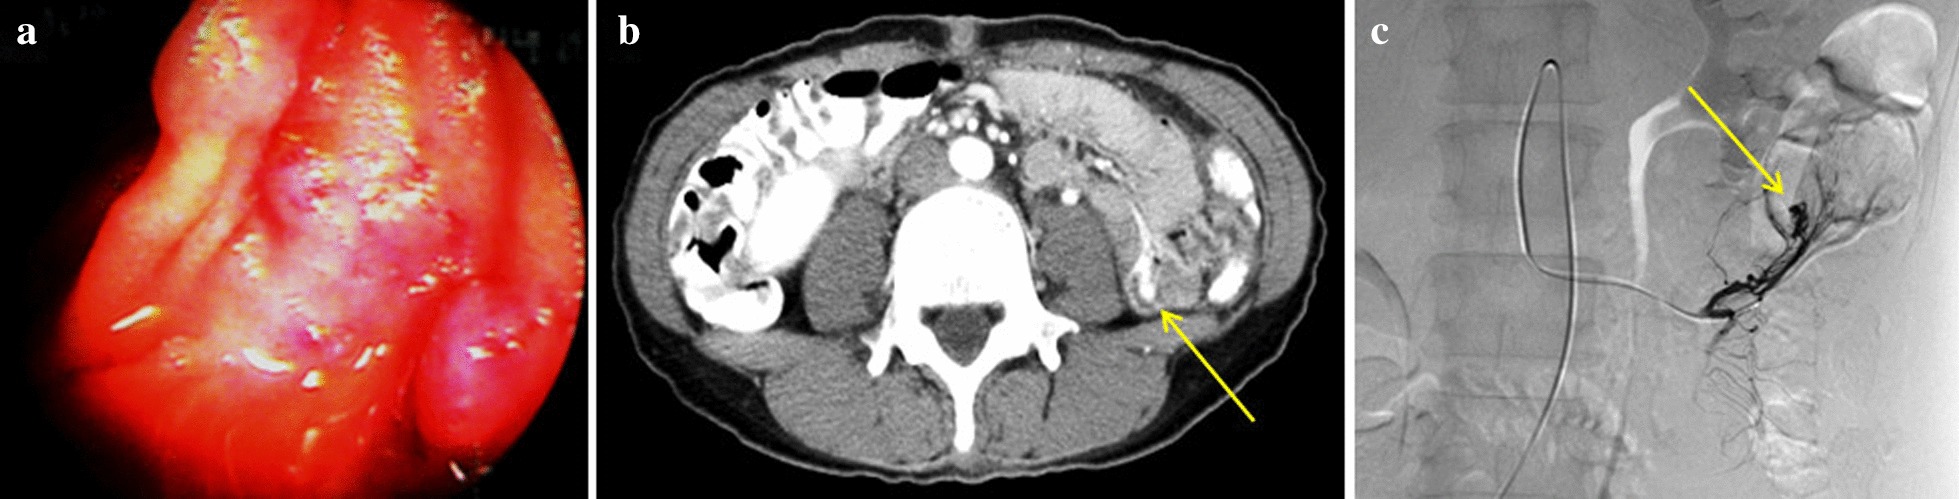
a Double-balloon endoscopy image. A vascular lesion is visible. b Contrast CT image. Arrow indicates contrast extravasation at the small intestinal wall. c Selective angiography images. Arrow indicates contrast extravasation peripheral to the first jejunal branch of the superior mesenteric artery
